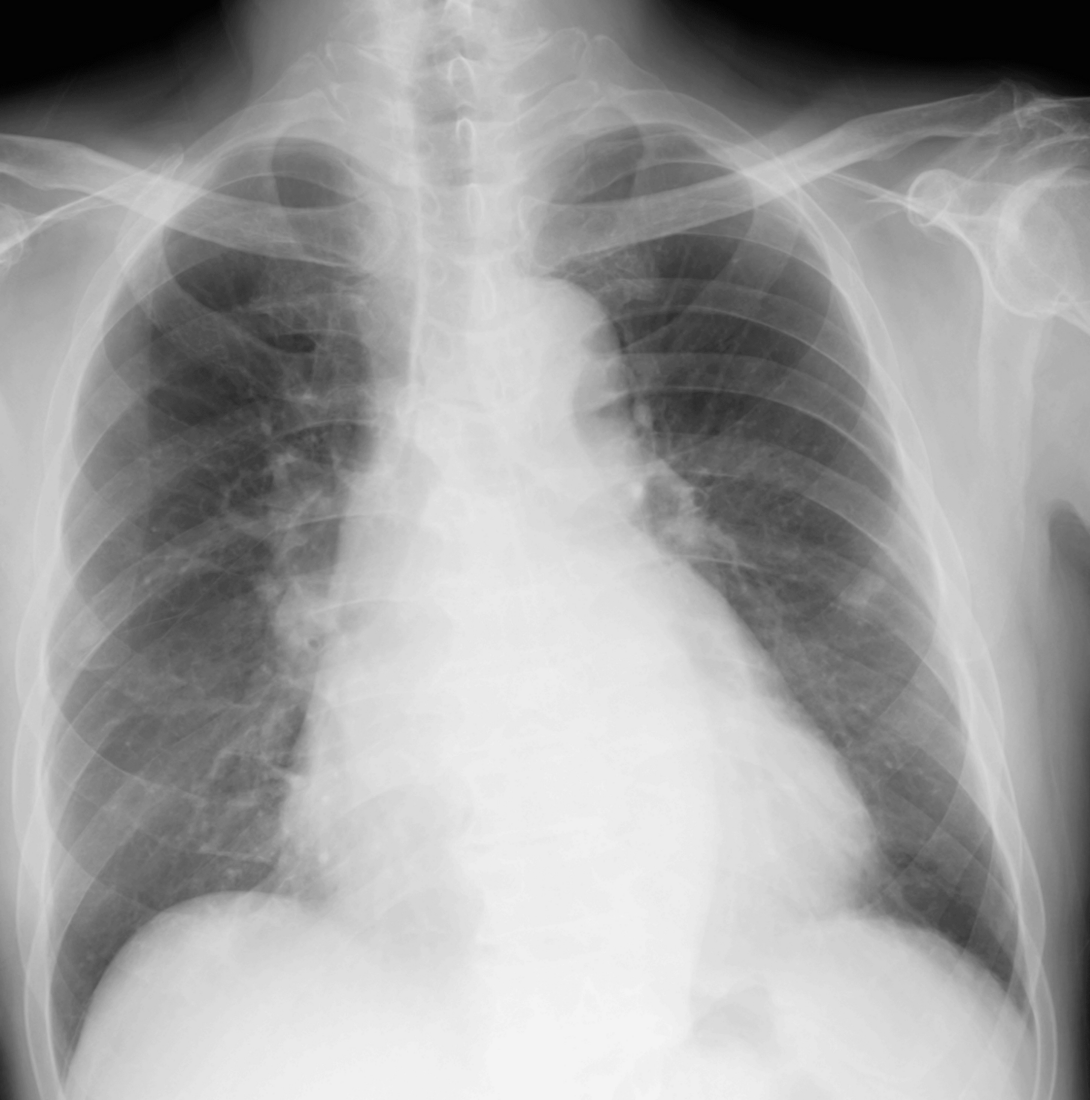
Preoperative AP chest radiograph. AP, anteriorposterior
